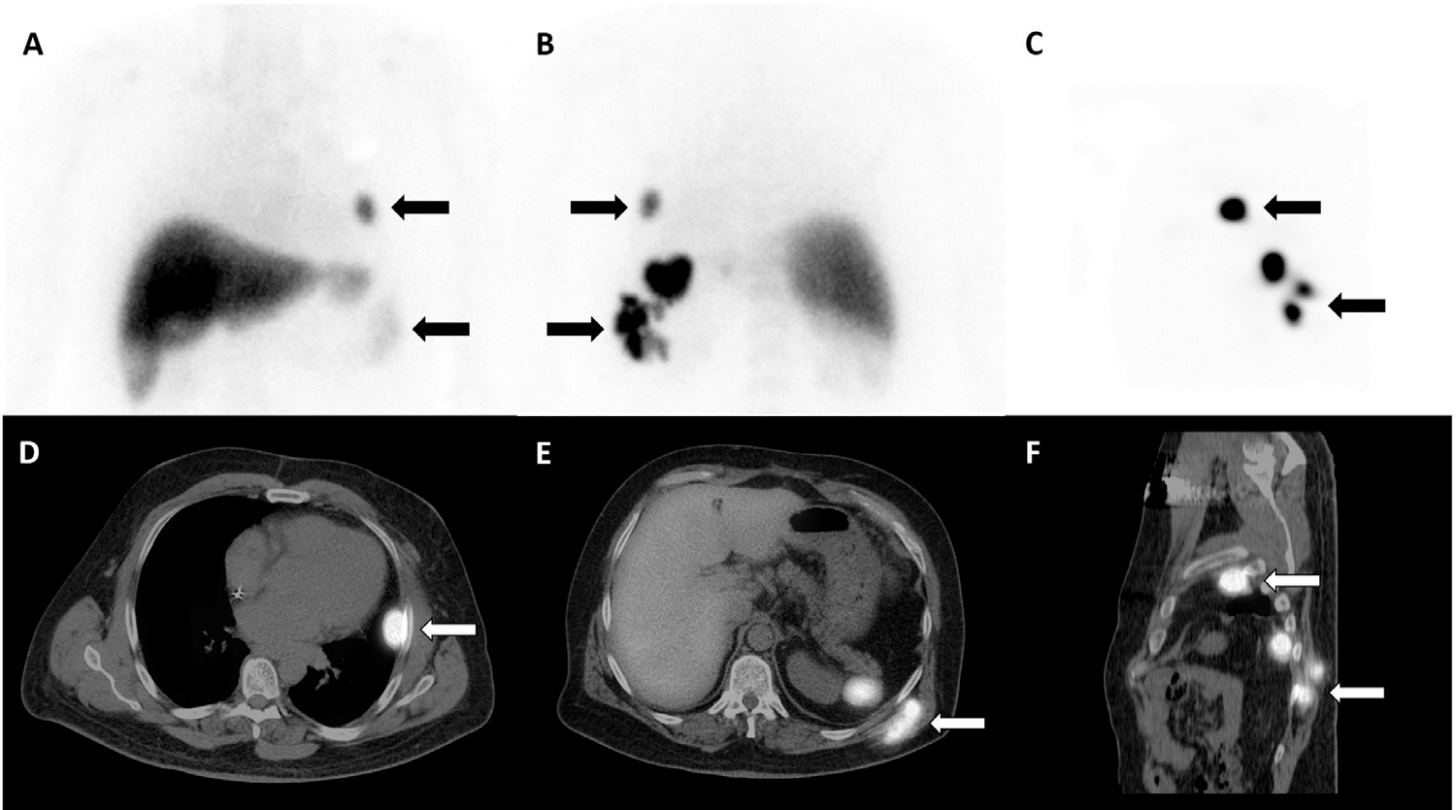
Technetium-99m labeled heat-damaged red cell scintigraphy anterior-posterior planar imaging and sagittal SPECT images demonstrated intense tracer activity in the region of the left hemithorax and left chest wall (A–C; Black arrows). Hybrid axial and sagittal SPECT/CT images confirmed extra-splenic tracer accumulation at the left pleural and left chest wall lesions, confirmed the diagnosis of combined thoracic and subcutaneous splenosis (D and E; White arrows).
